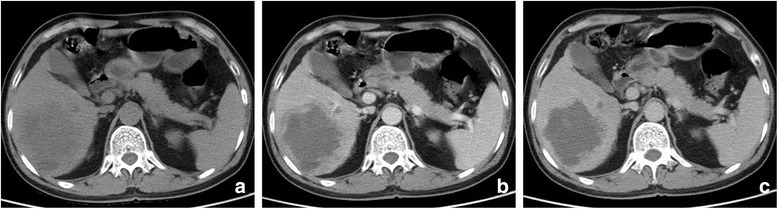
Computed tomography (CT) images of patient 5. a Unenhanced CT image reveal a hypodense multilobular mass in right lobe without a clear margin. Contrast enhanced CT reveals rim enhancement in AP (b) and washout in PP (c)
